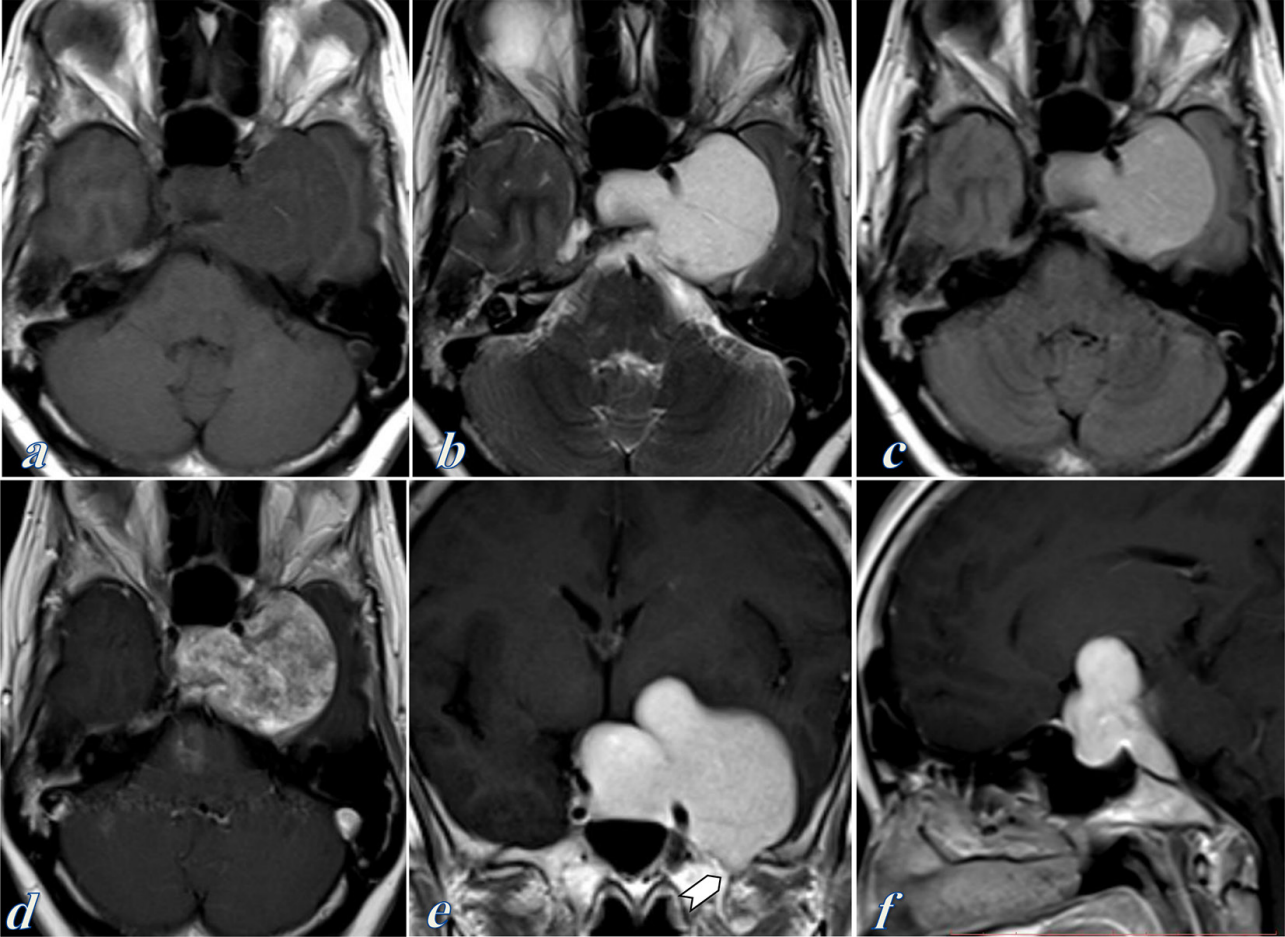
Contrast-enhanced MRI examination of the brain. (a) Pre-contrast axial *T*_1_ weighted image showed a large extra axial dumbbell sellar and left parasellar mass which elicits isointense to low signal intensity. (b, c) Axial *T*_2_ and FLAIR-weighted images showed remarkably high signal intensity of the mass. (d) Initial gadolinium-enhanced axial *T*_1_ weighted image showed heterogeneous enhancement of the mass. (e, f) Subsequent contrast-enhanced coronal and sagittal *T*_1_ weighted images showed intense homogeneous enhancement of the mass. The lesion causes enlargement of the left foramen ovale (Chevron in e). The cavernous internal carotid artery is mildly displaced laterally and is encased by the lesion without occlusion.
